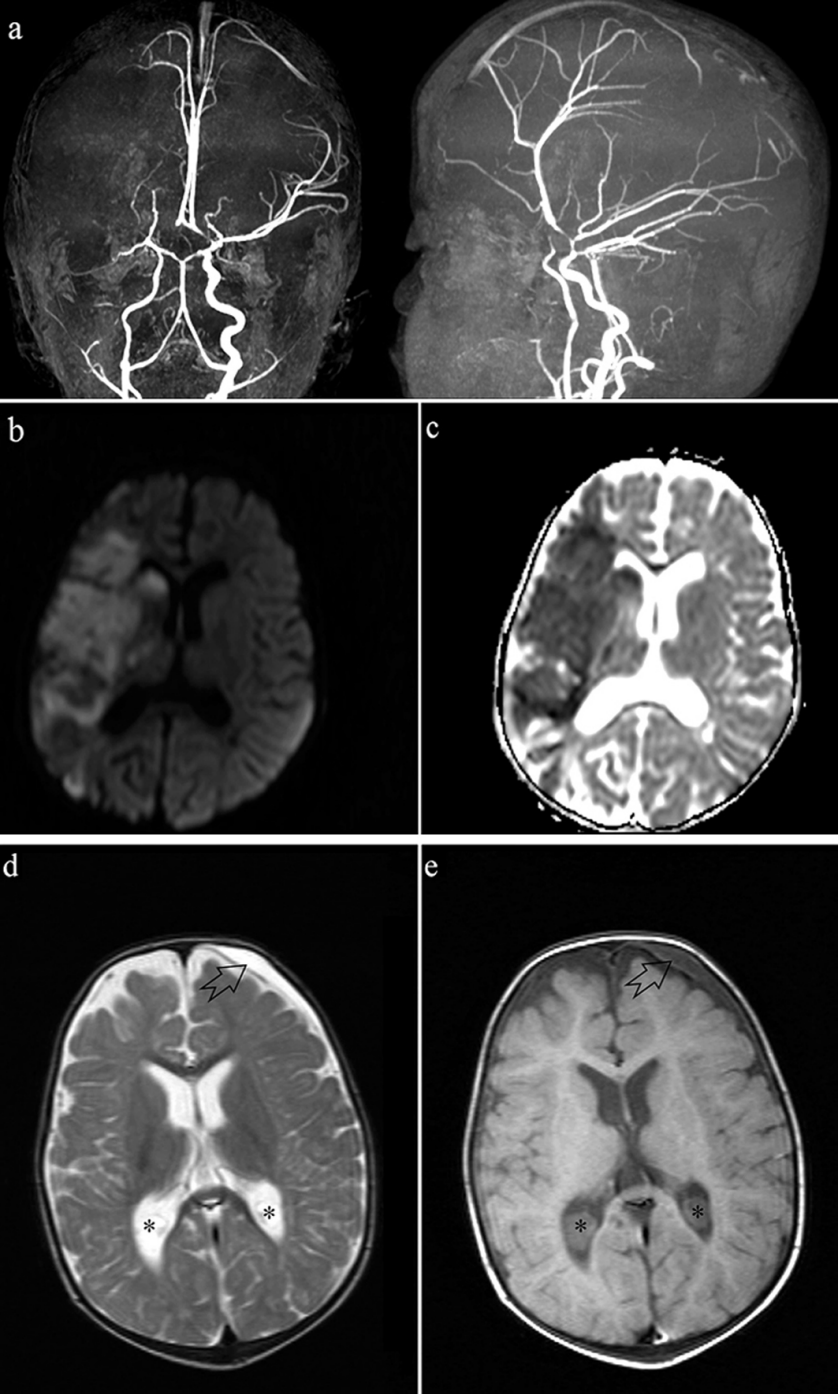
3-D MR angiography images in 2 planes (a) show the absence of right middle cerebral artery (MCA) and right anterior cerebral artery (ACA) flows. Other polygon arteries including the right carotid artery have irregular outlines and focal-segmental stenosis. Diffusion weighted imaging (DWI, B = 1000) (b) and apparent diffusion coefficient (ADC) (c) map images demonstrate a large acute infarction in the right MCA territory (One of 8-month-old twin siblings, MPS type I). Axial T2 (d) and T1 (e) weighted MR images reveal that there is an old subdural hemorrhage anterior to the left frontal lobe (black arrow). There are also bilateral choroid plexus cysts (asterisks) (16 months old, MPS type I).
